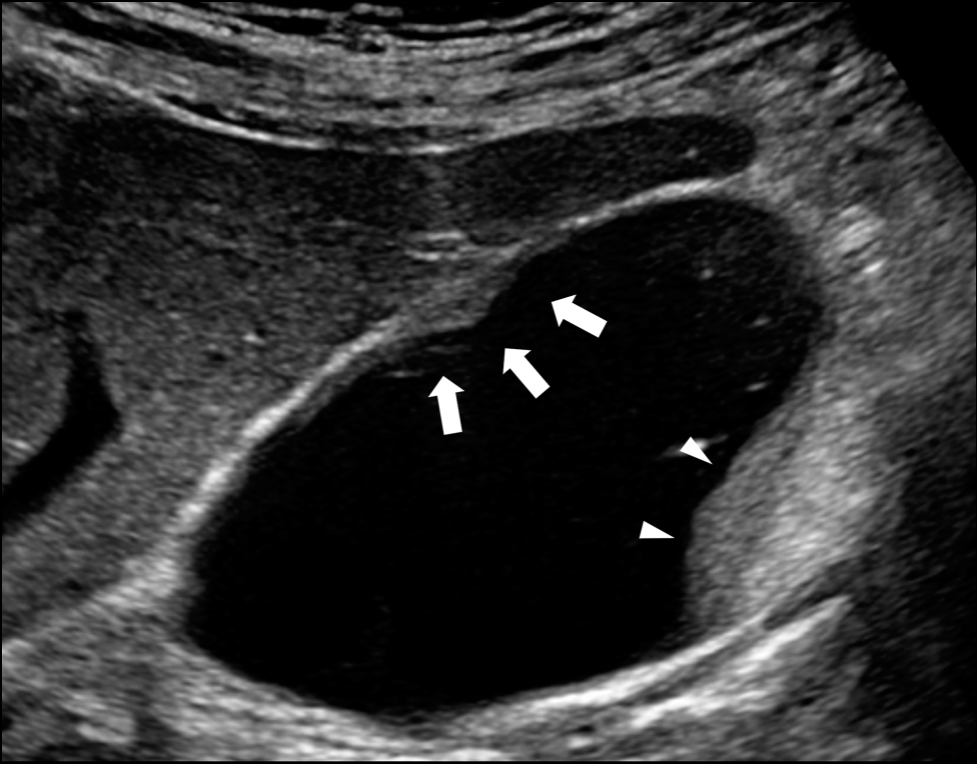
Abdominal ultrasonography. The gallbladder shows localized mural thickening (white arrows) with loss of a hyperechoic area at the liver-bed portion. Bile sludge (arrowheads) is observed.
